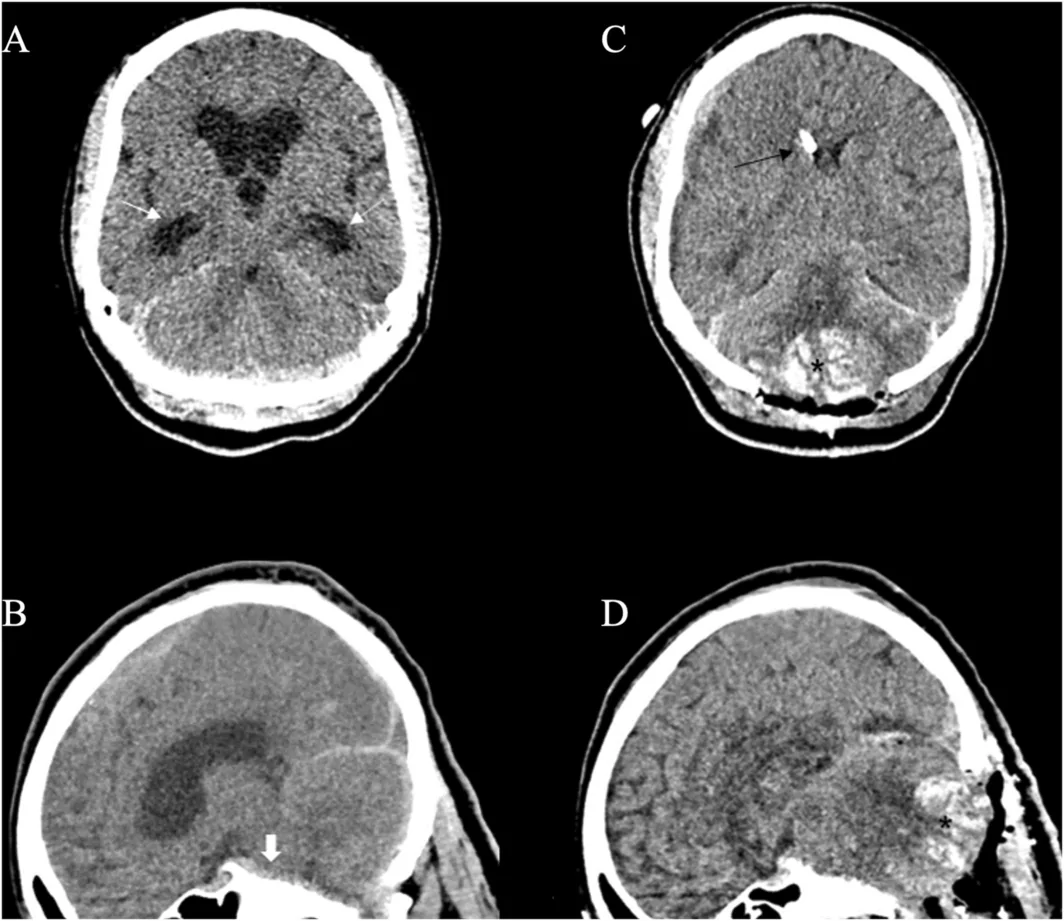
Cranial computed tomography (cCT). (A, B) Initial imaging with obstructive hydrocephalus due to infratentorial subarachnoid hemorrhage (SAH) (A white arrows: Temporal horn enlargement; B bold white arrow: SAH in the prepontine cisterna). (C, D) Imaging after posterior fossa decompression and external ventricular drainage (EVD) (C black arrow: Ventricular catheter tip; C, D asterisk: Cerebellar decompression hemorrhages).
